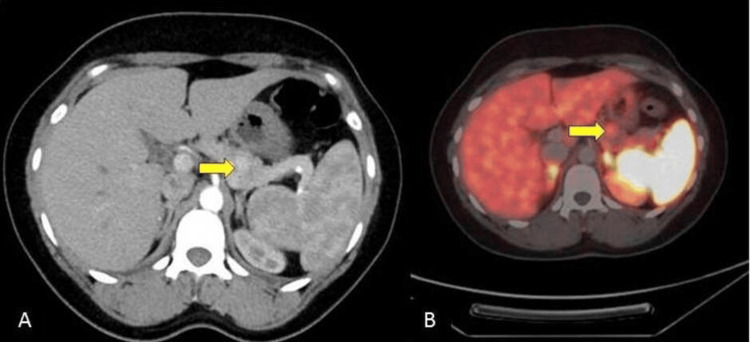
A: Contrast-enhanced CT of the abdomen showing 1.6x1.5x1.2 cm hyper-enhancing lesion in arterial phase in the distal body of pancreas (marked by arrow); B: Ga-DOTATATE scan showing an increased uptake by lesions in the distal body of pancreas suggestive of insulinoma (marked by arrow) At a standardized uptake value of 2.19
